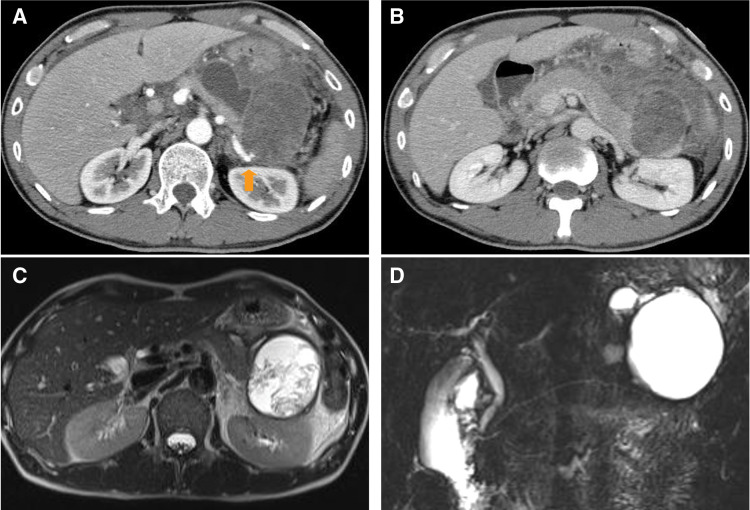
Imaging studies. (A) Contrast-enhanced CT scan showing a 7-cm heterogeneous cystic lesion with a pseudoaneurysm of the splenic artery in early-phase images (arrow). (B) Fluid collection with inflammatory changes was seen in late-phase images. (C) T2-weighted MRI showed a heterogeneous cystic lesion. (D) The main pancreatic duct was not dilated.
